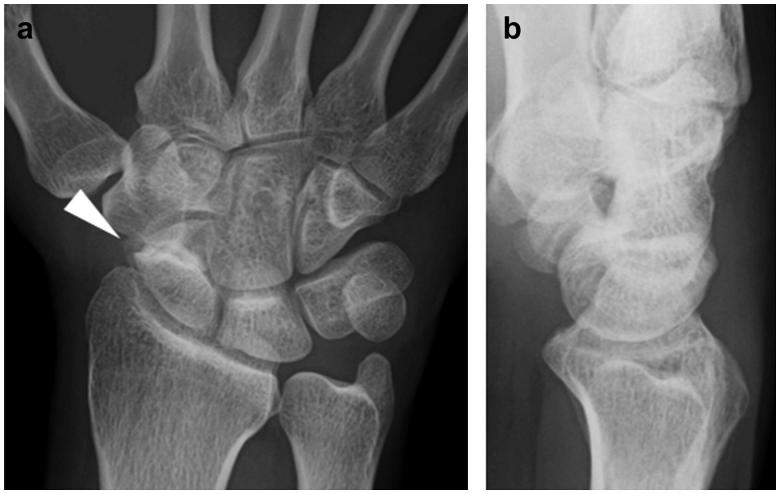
First radiographic assessment of the hand. (a) Posteroanterior and (b) lateral plain radiographs showing only scaphoid non-union.
